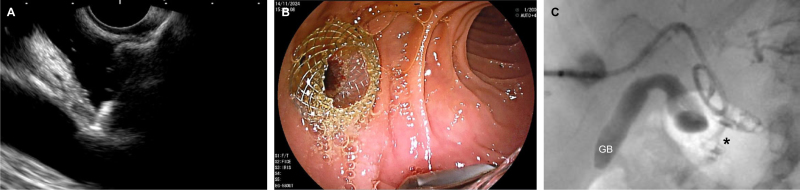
EUS-guided choledochoduodenostomy with lumen-apposing metal stent: distal flange opened on ultrasound view (A), proximal flange in good position on endoscopic view (B), confirmation of technical success on fluoroscopy (C). Asterisk shows position of the choledocho-bulbar metal stent; GB, gallbladder*.*
